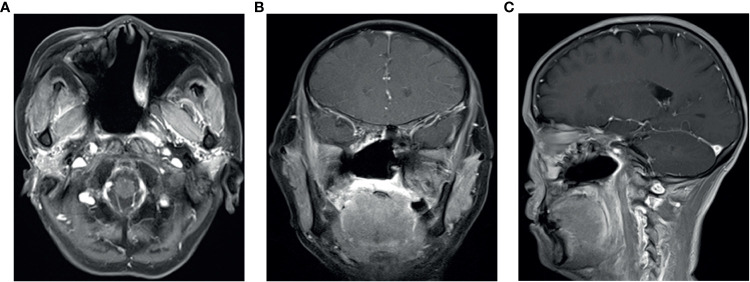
(A–C) In April 2020, Nasopharyngeal MRI showed that there was no local recurrence after radiotherapy and anlotinib treatment. Figures A–C show the local situation from transverse, coronal and sagittal positions, respectively.
